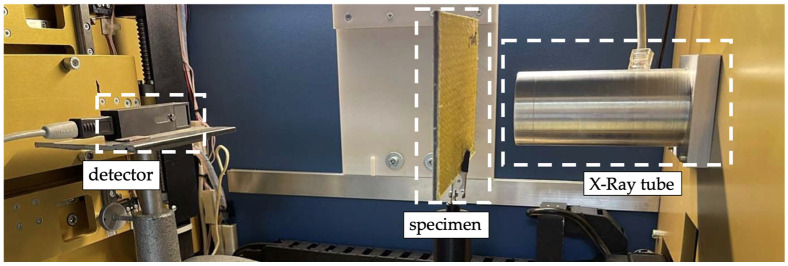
A scheme of the inspection process using the laboratory radiographic setup.
